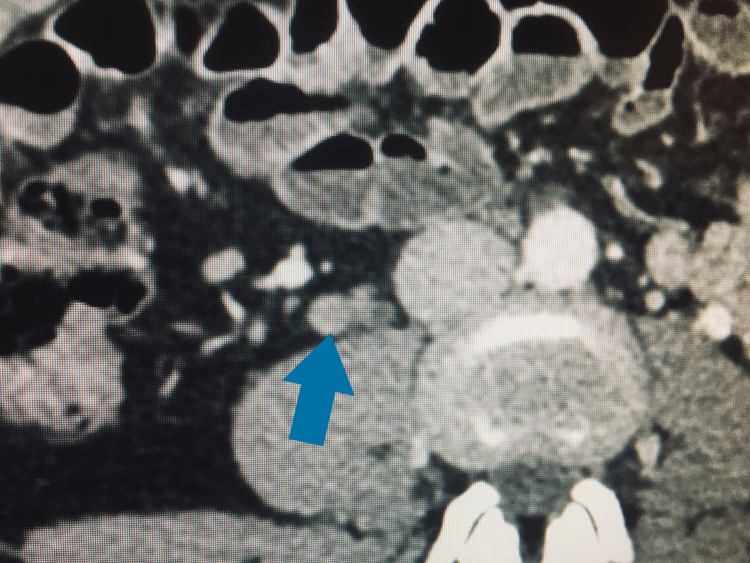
Follow-up CT scan showing an axial view of the ovarian vein (blue arrow) There is no filling defect. Vein diameter is smaller.
